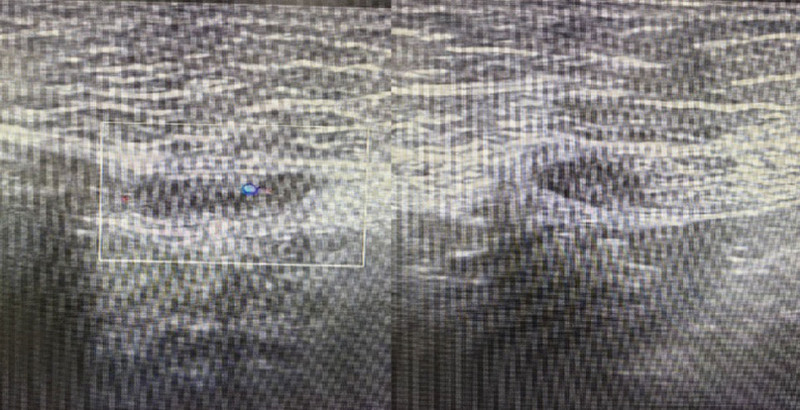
Ultrasound of abdominal wall showed a hypoechoic mass within the muscle layer of the lower left abdomen, measuring approximately 12 mm × 8 mm.
